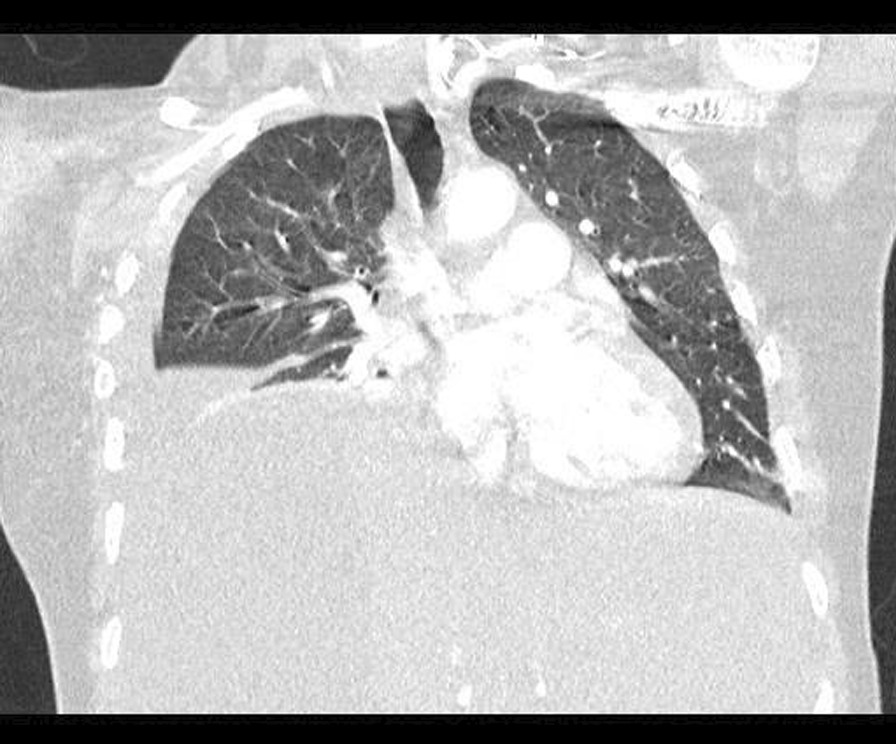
Computed tomography chest postoperative day 15: Large right sided pleural effusion with mediastinal shift.
